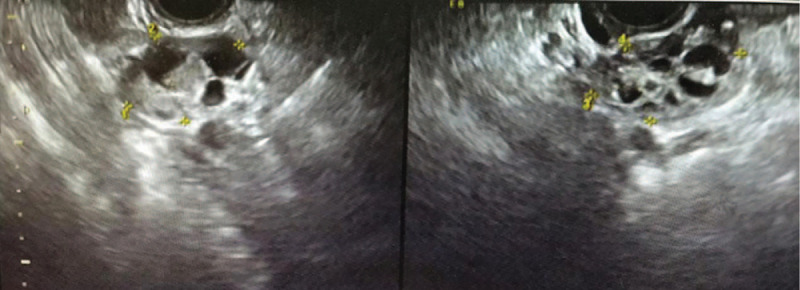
Vaginal ultrasound showing that the maximum diameters of the lumps in the right and left ovaries were 46 mm × 34 mm and 46mm × 29 mm respectively, which were also cystic and solid components.
